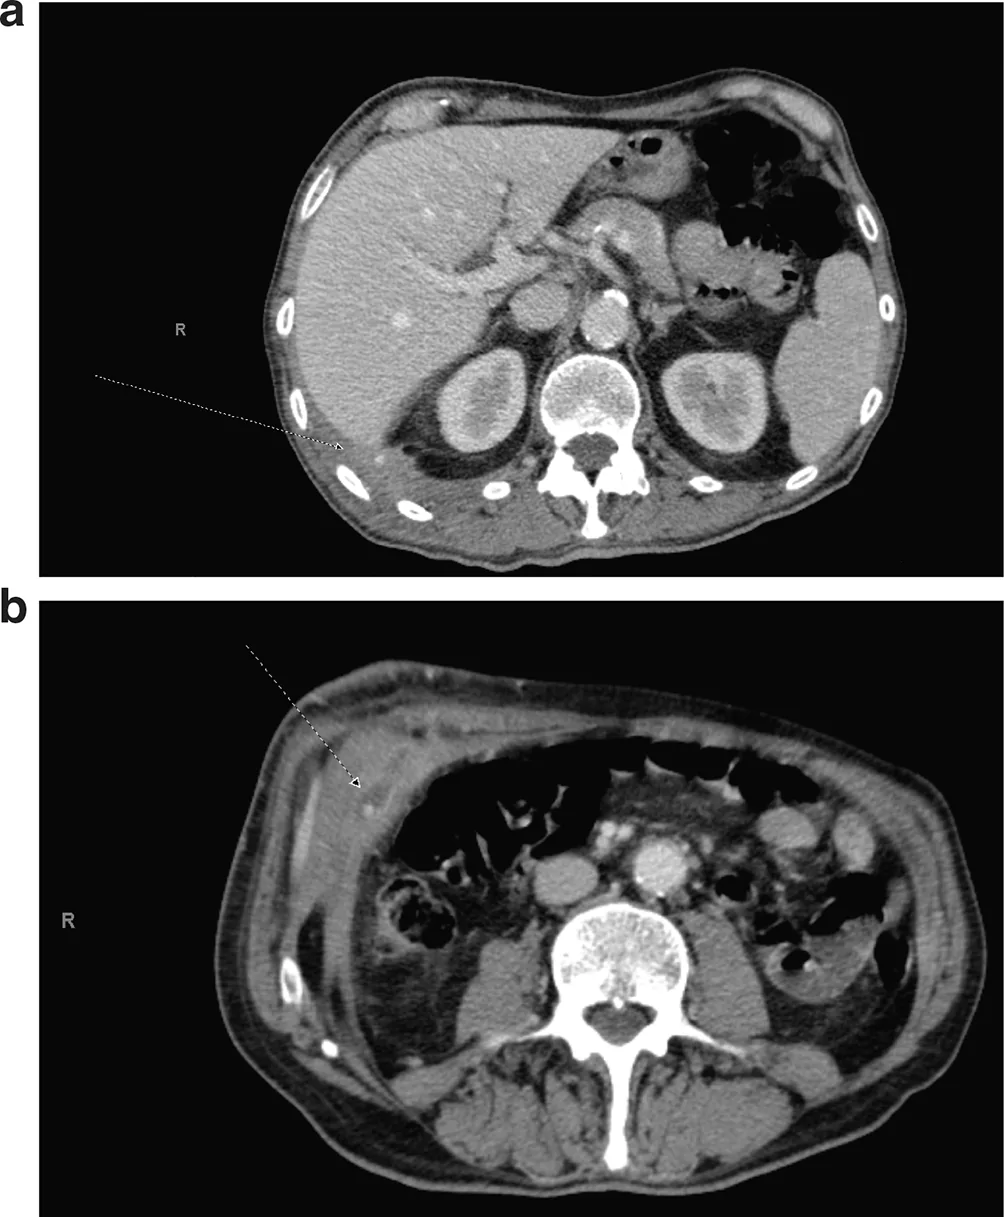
Abdominal computed tomography. An abdominal computed tomography showing spilled gallstones at different levels 15 months after the laparoscopic cholecystectomy (dotted arrows). (a) Shows a gallstone behind the liver and (b) shows a gallstone in the abdominal wall.
